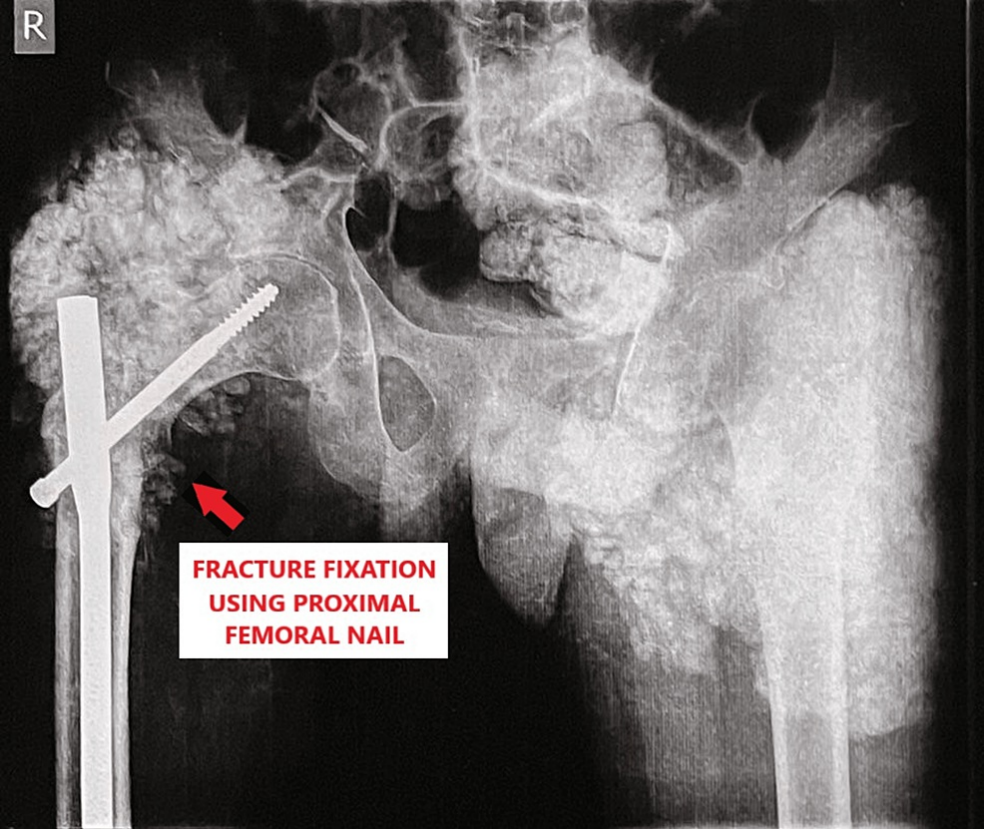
Post-operative radiograph showing fracture fixation using a proximal femoral nail.
